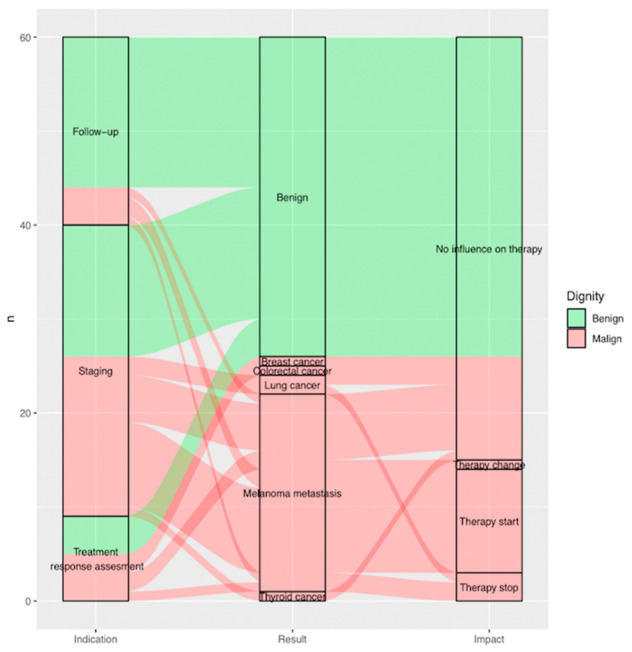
Sankey plot showing, per included indeterminate finding (*n* = 60), on the far left, (a) indication of FDG-PET/CT scan it was reported on as indeterminate; in the middle, (b) result of additional imaging; and on the far right, (c) impact of additional imaging on clinical management. Benign findings are displayed in green, malignant findings in red arrows. (a) Indication (on the left from the bottom to the top): treatment response assessment (*n* = 9; 15.0%), staging (*n* = 31; 51.7%) and follow-up (*n* = 20; 33.3%). (b) Result (in the middle from the bottom to the top): thyroid cancer (*n* = 1; 1.7%), melanoma metastasis (*n* = 21; 35.0%), lung cancer (*n* = 2; 3.3%), colorectal cancer (*n* = 1; 1.7%), breast cancer (*n* = 1; 1.7%), benign (*n* = 34; 56.7%). (c) Impact *(*on the right from the bottom to the top): therapy stop (*n* = 3; 5.0%), therapy start (*n* = 11; 18.3%), therapy change (*n* = 1; 1.7%) and no influence on therapy (*n* = 45; 75%).
